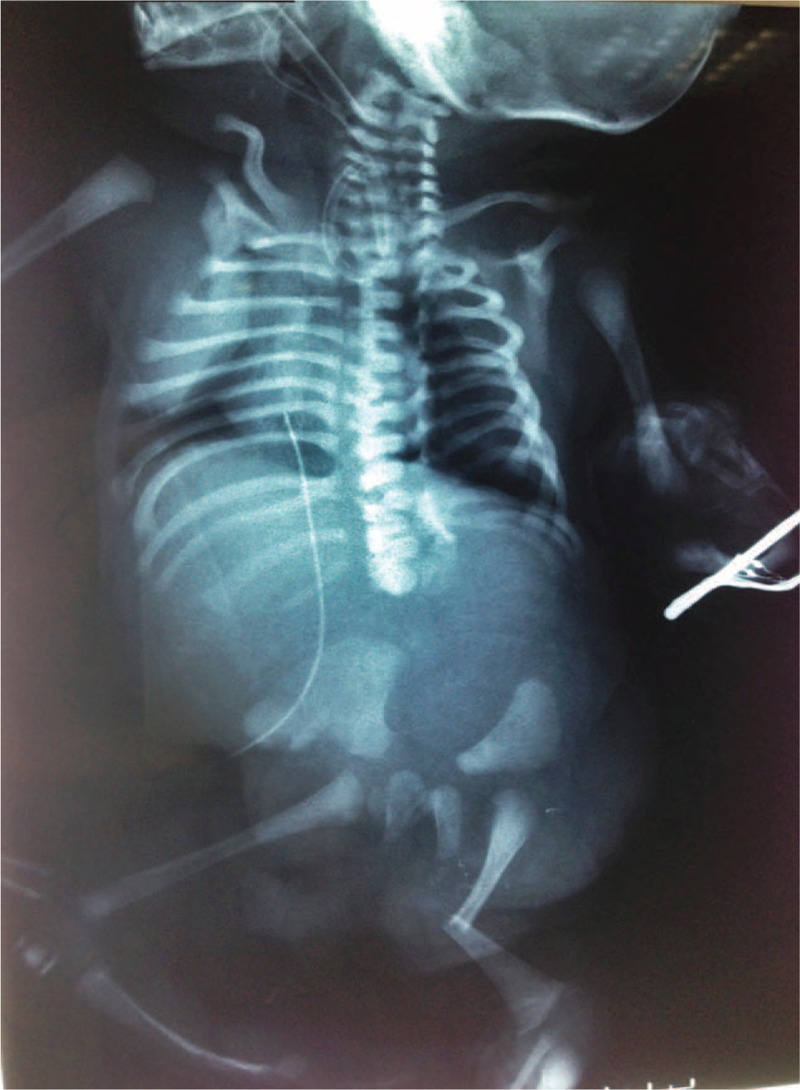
Radiograph of case 9 shows absence of lumbosacral vertebrae, thoracic vertebral defects, malformed ribs, dysplastic and hypoplastic pelvic bones, and “frog-like” position of the right lower extremity. Spine terminates at the T12 level. The left radius is absent (the absence of left thumb is not well visualized). The orogastric tube does not pass through esophagus, and is twisted. There is no passage of gas into the stomach and intestines. Note shaft fracture of the left femur, and dislocated left knee.
